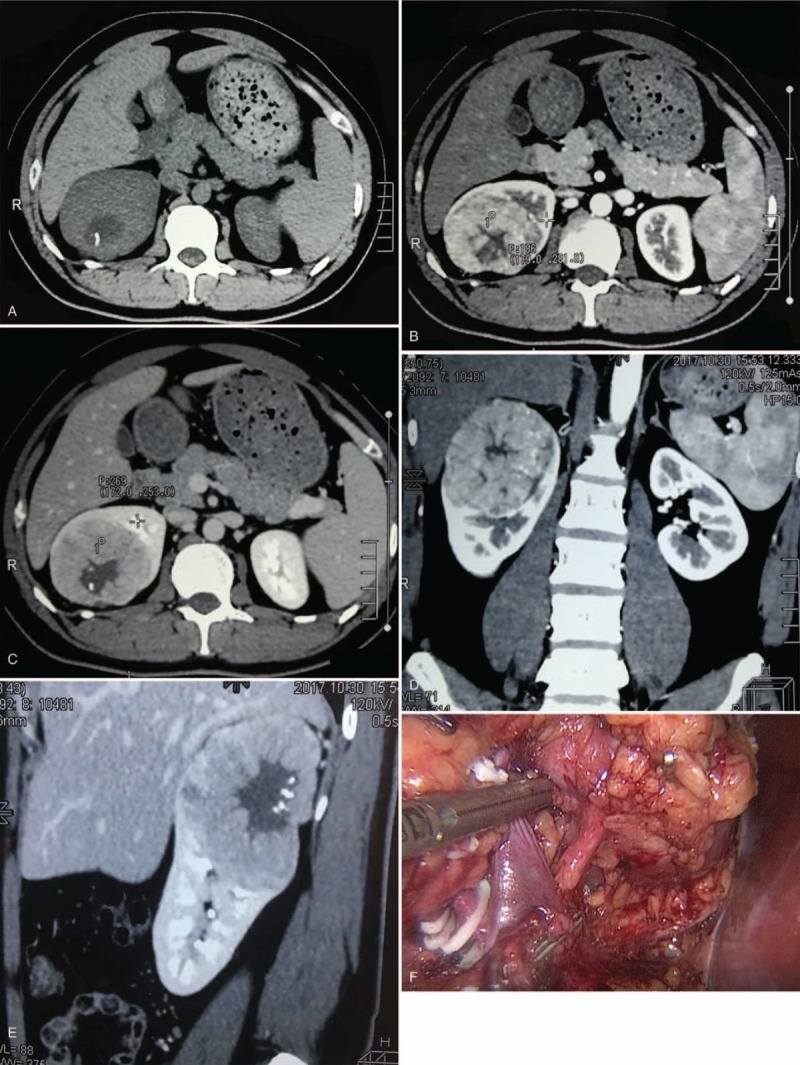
Preoperative CT and intraoperative imaging. A, Plain scan shows a large mass measured 8.5 cm in diameter in the upper pole of the right kidney, displaying central stellate hypodensity areas inside with punctate calcification. B, The mass has an apparently enhancement in arterial phase. C, The mass shows a relatively low density compared with parenchyma in venous phase. D, The mass in contour view. E, The mass in sagittal view. F, Laparoscopic right radical nephrectomy was performed and the mass is found to have been supplied by independent artery. CT = computed tomography.
